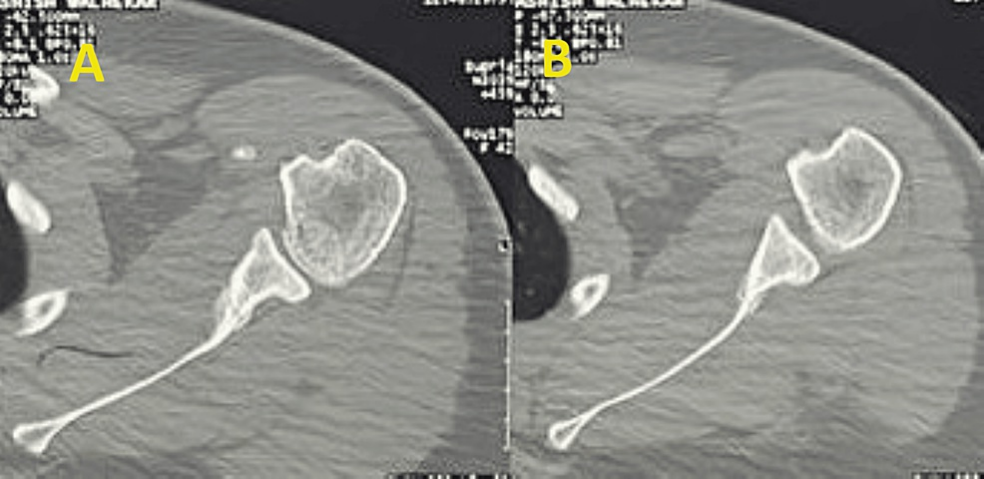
Post 20-week follow-up CT scan showing good callus formation and no further displacement A)-B) Serial axial CT cuts of the left shoulder joint
